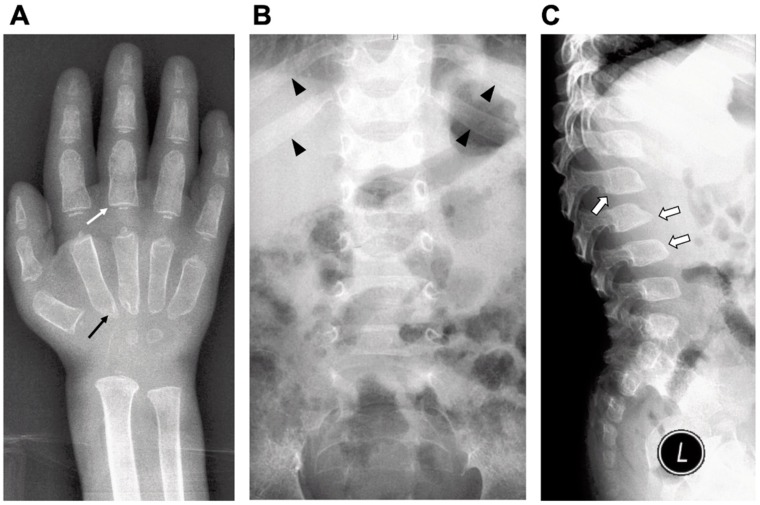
Radiographic findings of a MPSPS patient at age of one-year and nine-month. Characteristics of dysostosis multiplex: (A) bullet-shaped phalanges (white arrow) and the metacarpal pointing (black arrow); (B) widening of anterior ribs (oar-shaped ribs) (arrowhead) and (C) vertebral dysplasia with round- and hooked-shape vertebral bodies (open arrow).
